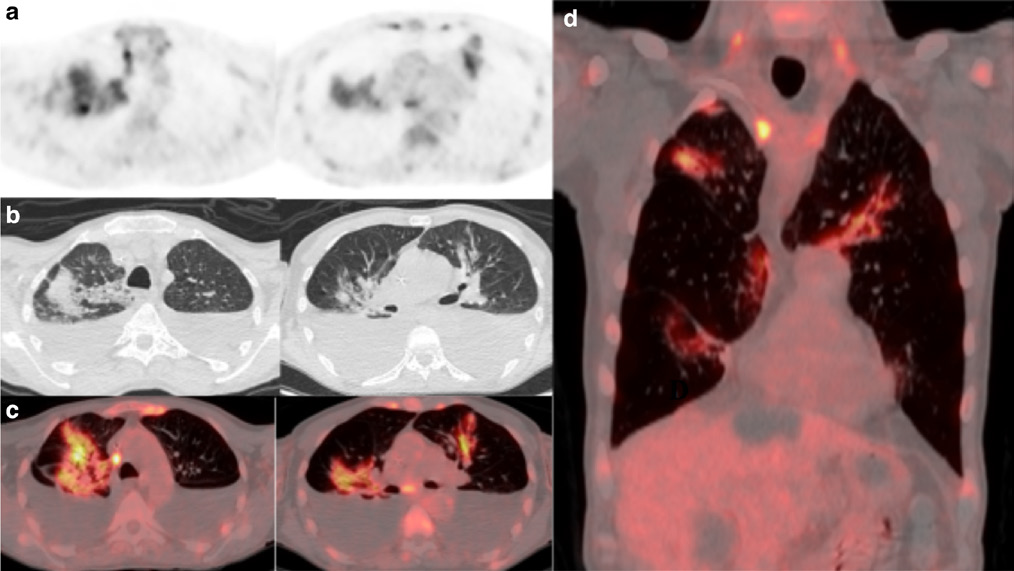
(a) ^18^FDG-PET thorax, (b) axial low-dose CT thorax, (c) axial fused thoracic ^18^FDG-PET/CT, (d) coronal fused thoracic ^18^FDG-PET/CT. The images demonstrate hypermetabolic patchy perihilar consolidation and GGO in three lobes and avid mediastinal lymph nodes. ^18^FDG-PET, 18-fludeoxyglucose-positron emmision tomography; GGO, ground glass opacity.
